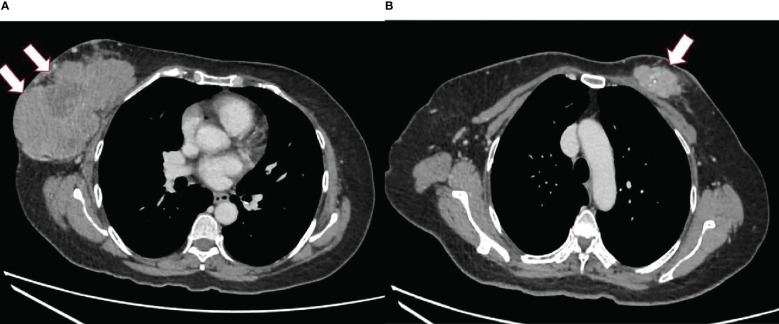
CT scan of the thorax. (A) Right breast mass, arrowed. (B) Left breast mass, arrowed.
